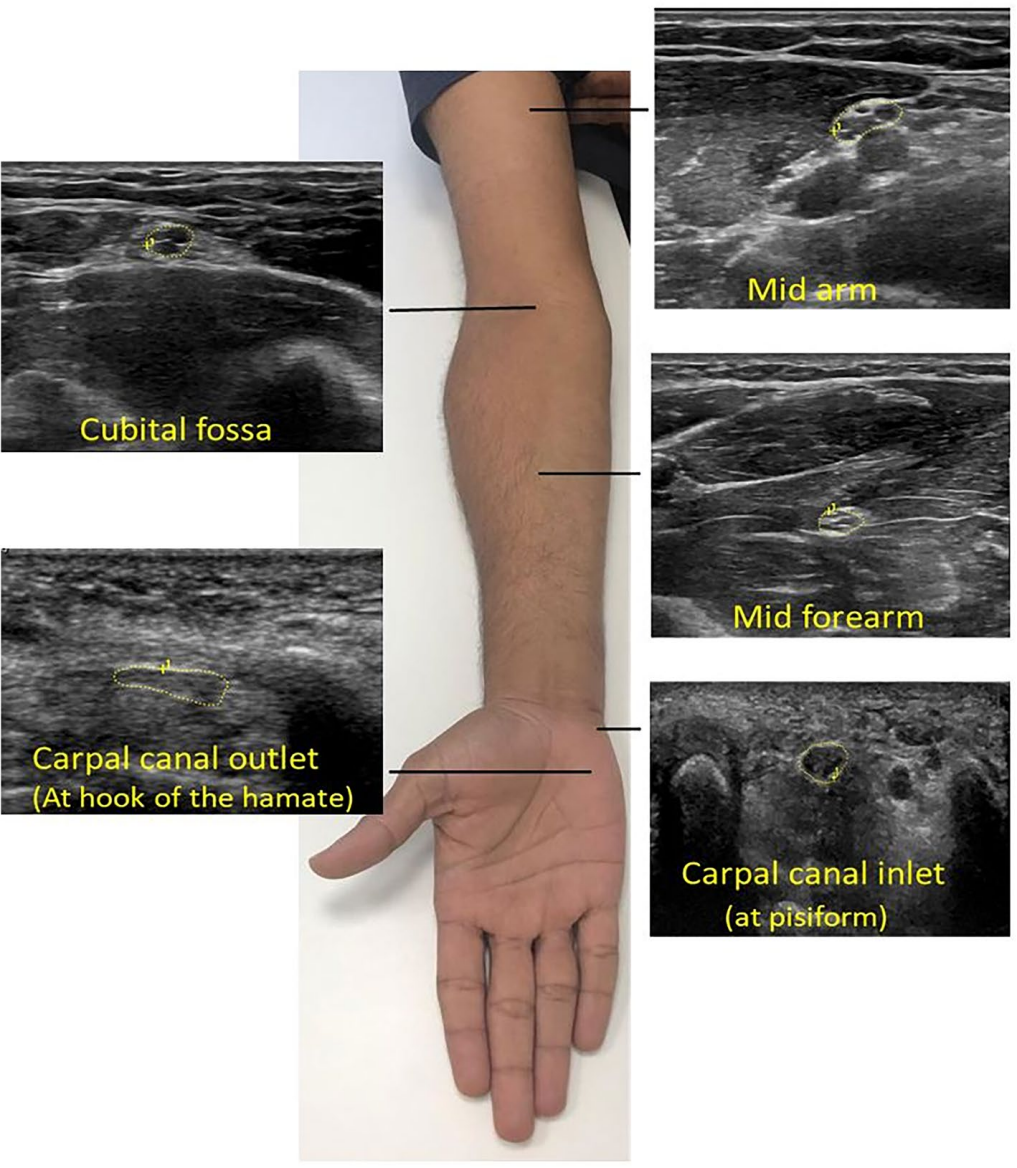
Ultrasound images and direct tracing of the median nerve cross sectional area at five different points of the right upper limb. A High-frequency 6–5 MHz linear array transducer was used (Logiq Eg, GE healthcare, WI, USA).
